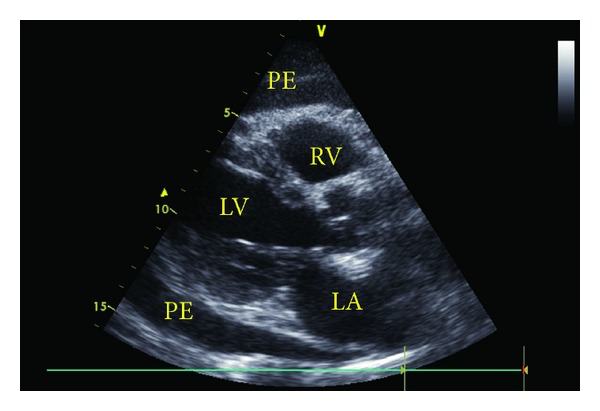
Parasternal long axis view with transthoracic echocardiography demonstrating pericardial effusion (PE: pericardial effusion, RV: right ventricle, LV: left ventricle, and LA: left atrium).
